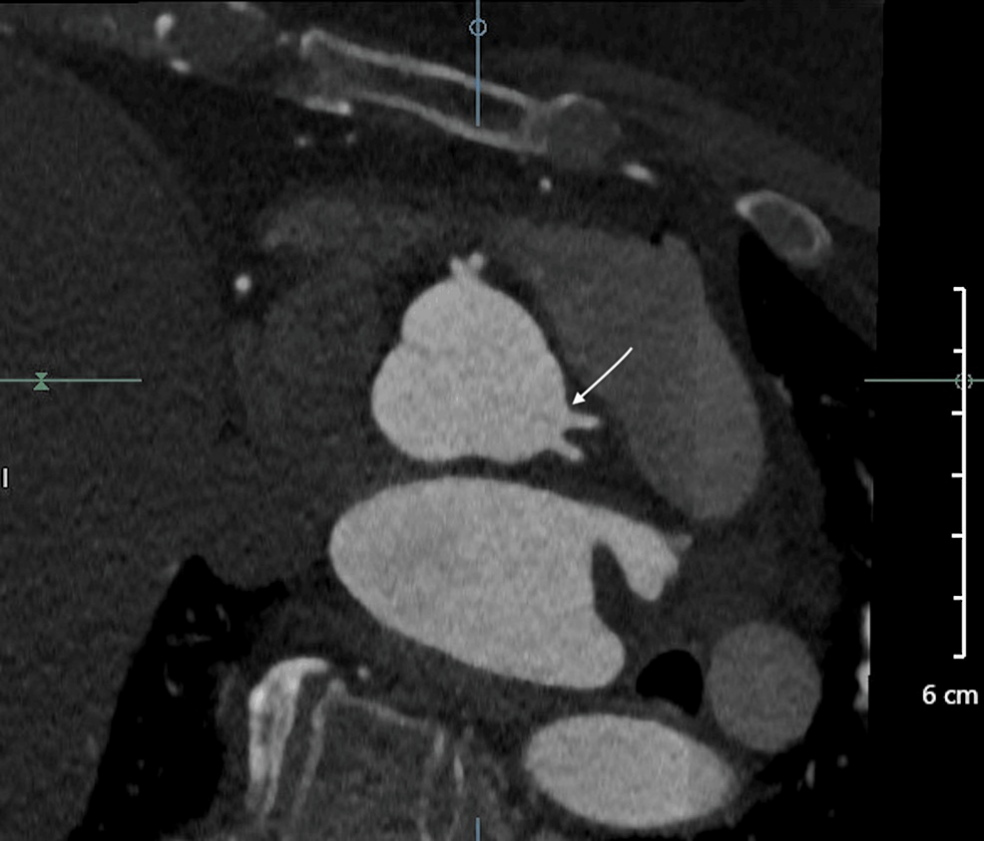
CTA with a white arrow showing the separate ostia arising from the left sinus of Valsalva that is giving rise to a short LAD and LCx CTA: computed tomography angiography; LAD: left anterior descending; LCx: left circumflex
